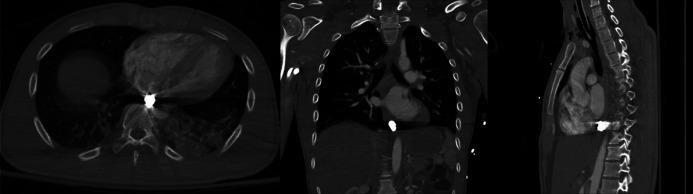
Computed tomography imaging of swallowed bullet.
